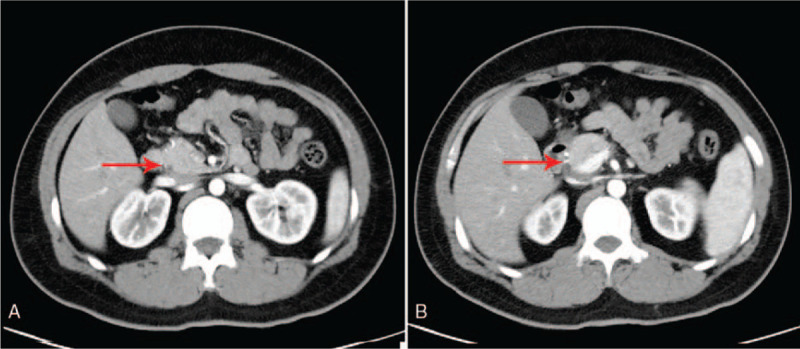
Abdominal enhancement computed tomography scan: Red arrows indicate a homogenous mass measuring 7.1 cm × 7.6 cm with uniform enhancement.
